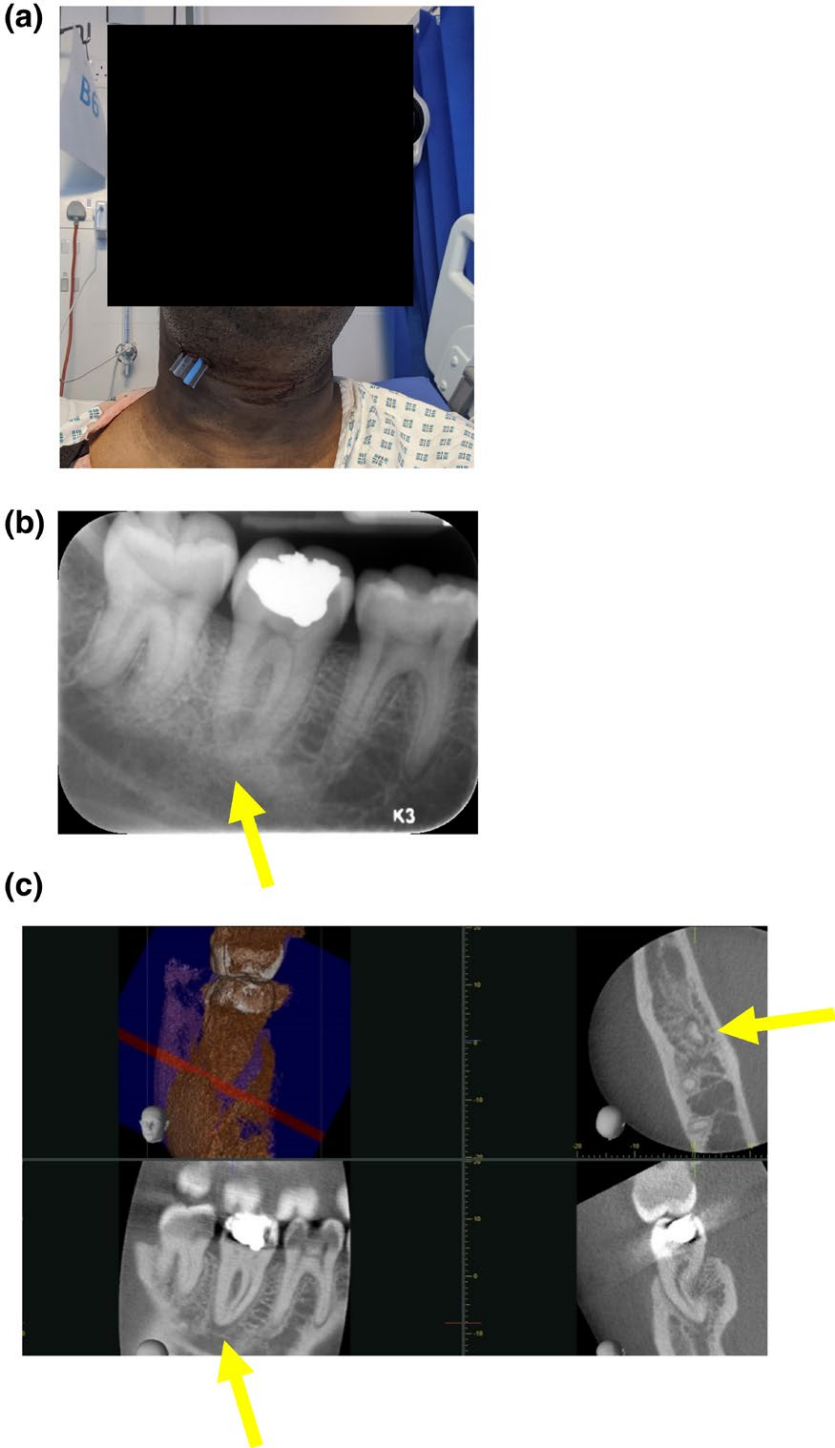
(a) The pulp necrosis of the lower right second molar (LR7) has caused a large abscess with cellulitis requiring extra‐oral drainage. (b, c) The periapical radiograph does not show any obvious radiolucency and the CBCT scan shows the presence of a 2 mm apical radiolucency associated with the LR7 (indicated by the yellow arrow).
